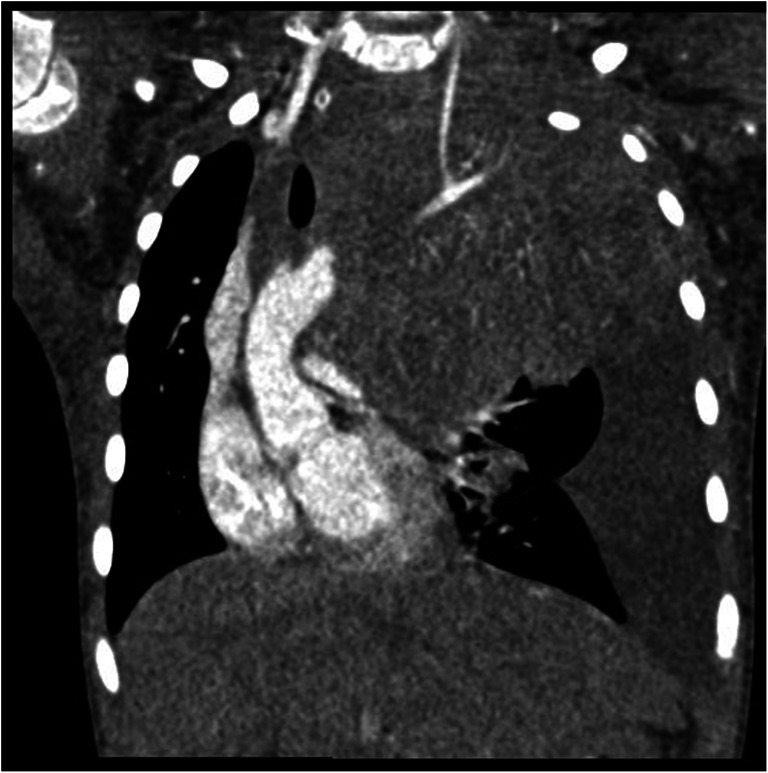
CT of patient with a NBL tumor involving aorta, heart, trachea and left bronchi
